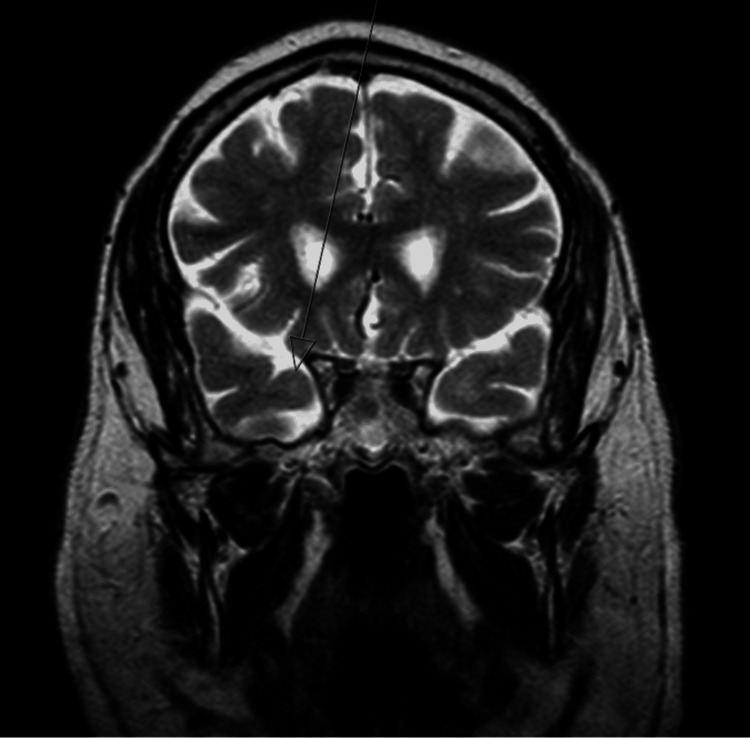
Brain MRI coronal T2 showing hyperintensity in the right anterior temporal lobe
